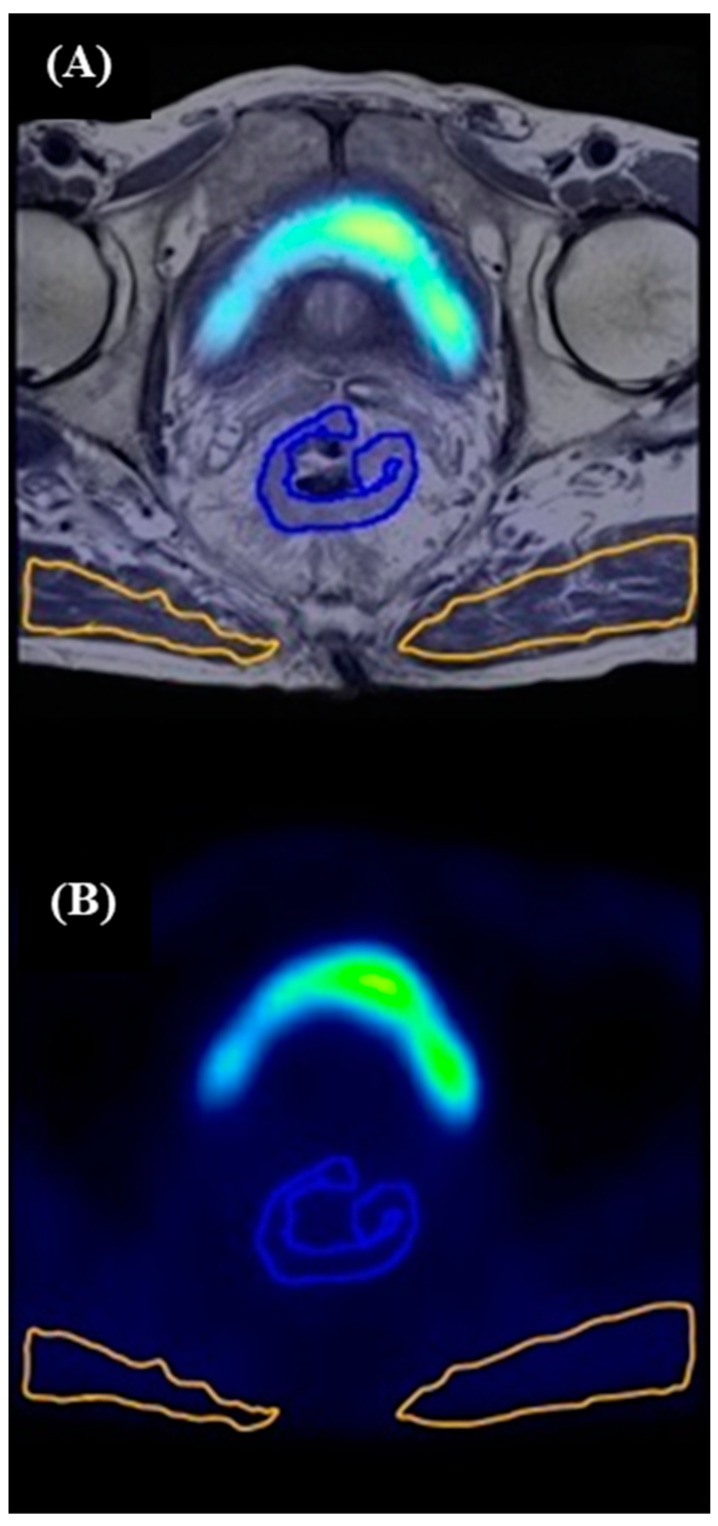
Segmentation method guided with T_2_-weighted MR image fused with ^18^FAZA PET image (A) and corresponding ROI in ^18^F-FAZA PET image alone (B). Segmentation method. Axial T_2_-weighted MR image at the level of mid-rectum with tumor segmented (blue contour) and gluteus maximum muscle segmented bilaterally (orange contour).
